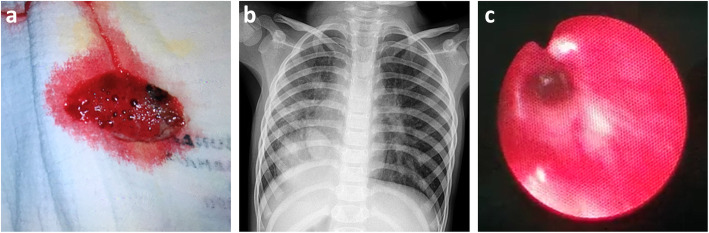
a Expectorant consisted of fresh bright blood mixed with small clots and mucus. b Chest radiograph showing ambiguous situs (abnormal liver and stomach position), mesocardia and a mass over the right para-cardiac region. c Bronchoscopic image of right main bronchus showing mucosal hyperaemia, oedema and variceal engorgement of submucosal blood vessels
